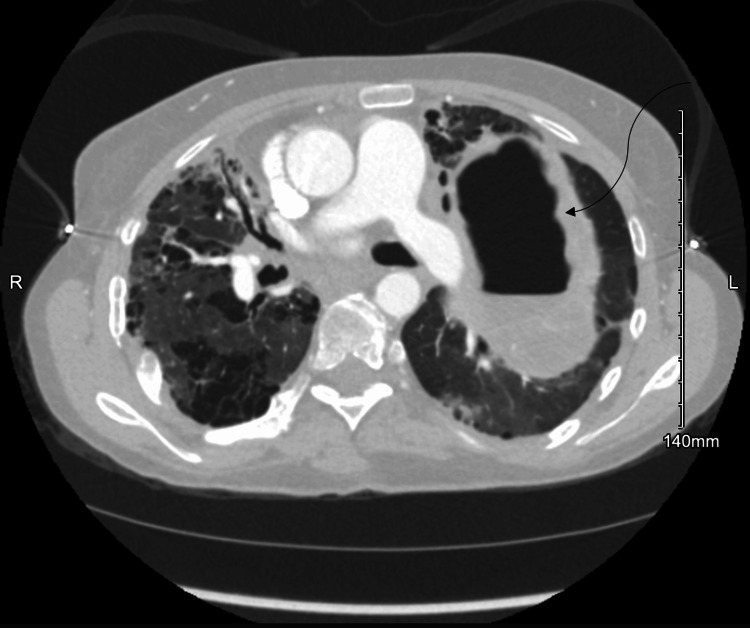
Computed Tomography Scan Demonstrating a Large Left Upper Lobe Cavitary Lesion With Surrounding Infiltrates The image shows a de-identified left upper lobe cavitary lesion in a patient with *STAT3*-deficient hyperimmunoglobulin E syndrome and secondary hypogammaglobulinemia. No identifying patient information is present, and informed consent for publication was obtained.
